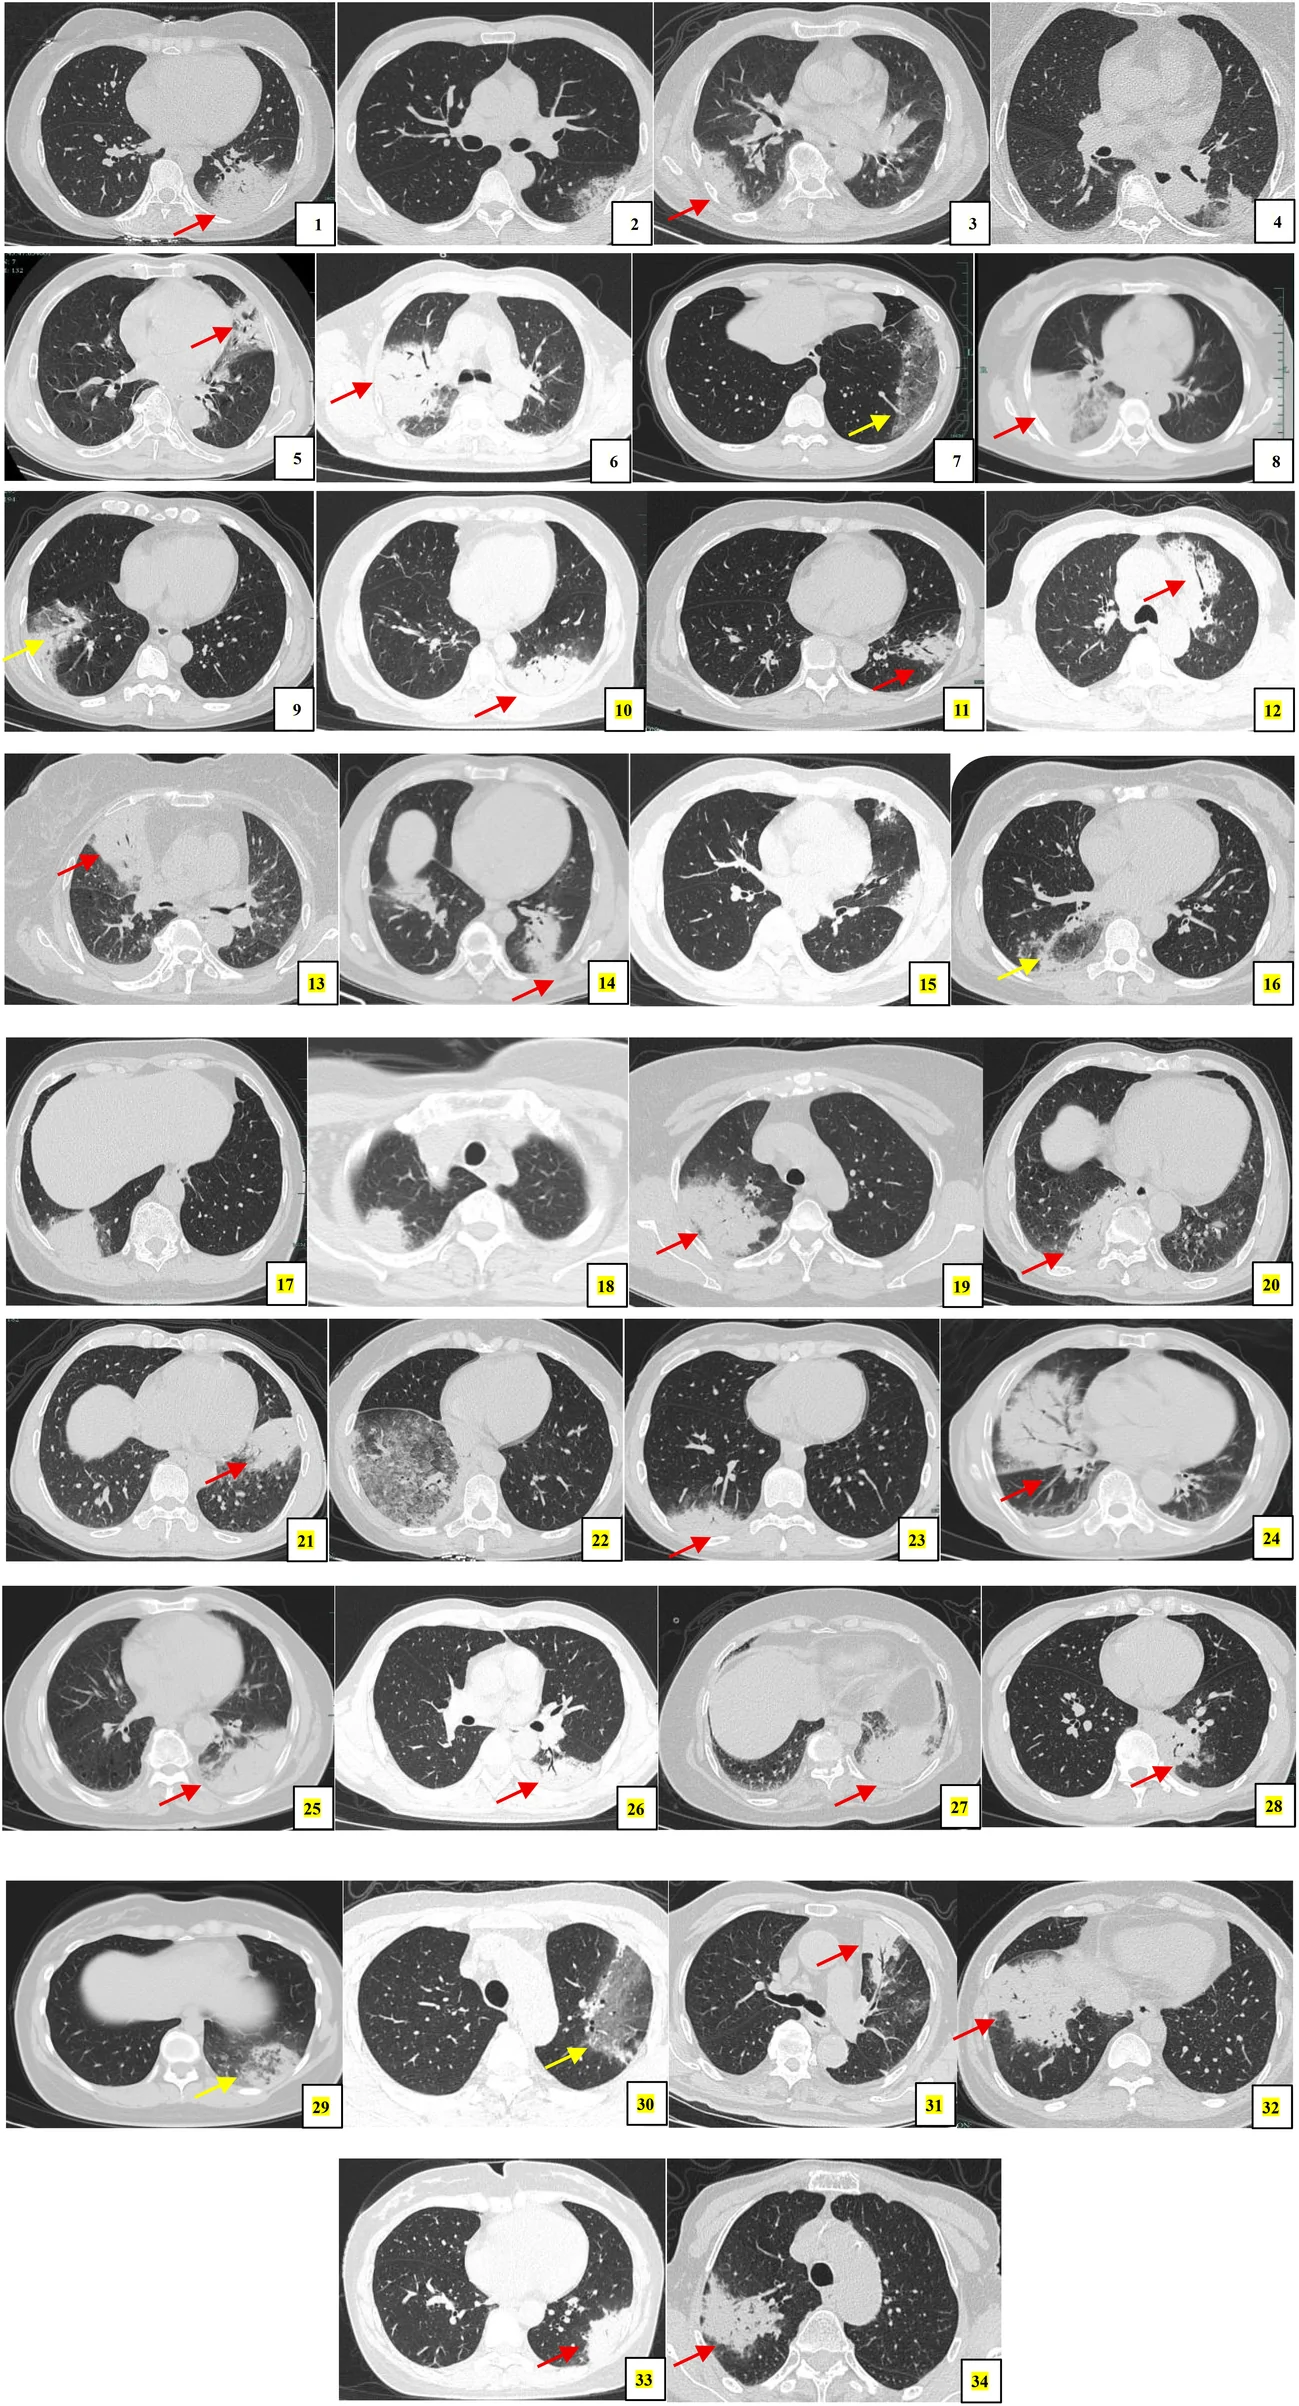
Chest CT scans of 34 patients with *C. psittaci* pneumonia.
